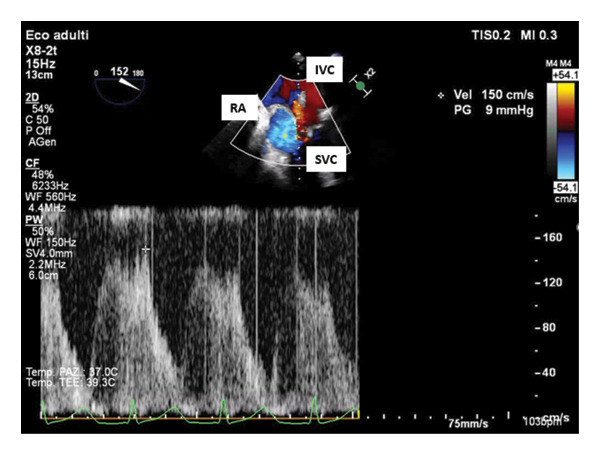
Deep transgastric pulsed wave color Doppler flow velocity in the acute superior vena cava stenosis. RA: right atrium; IVC: inferior vena cava; SVC: superior vena cava.
